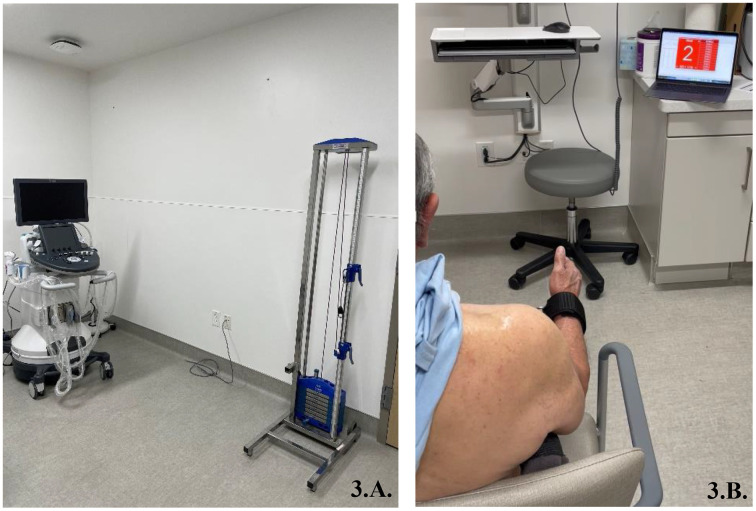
A. Ultrasound shear wave elastography and pulley weight system setup. B. Patient performing the external rotation isometric exercise protocol.
